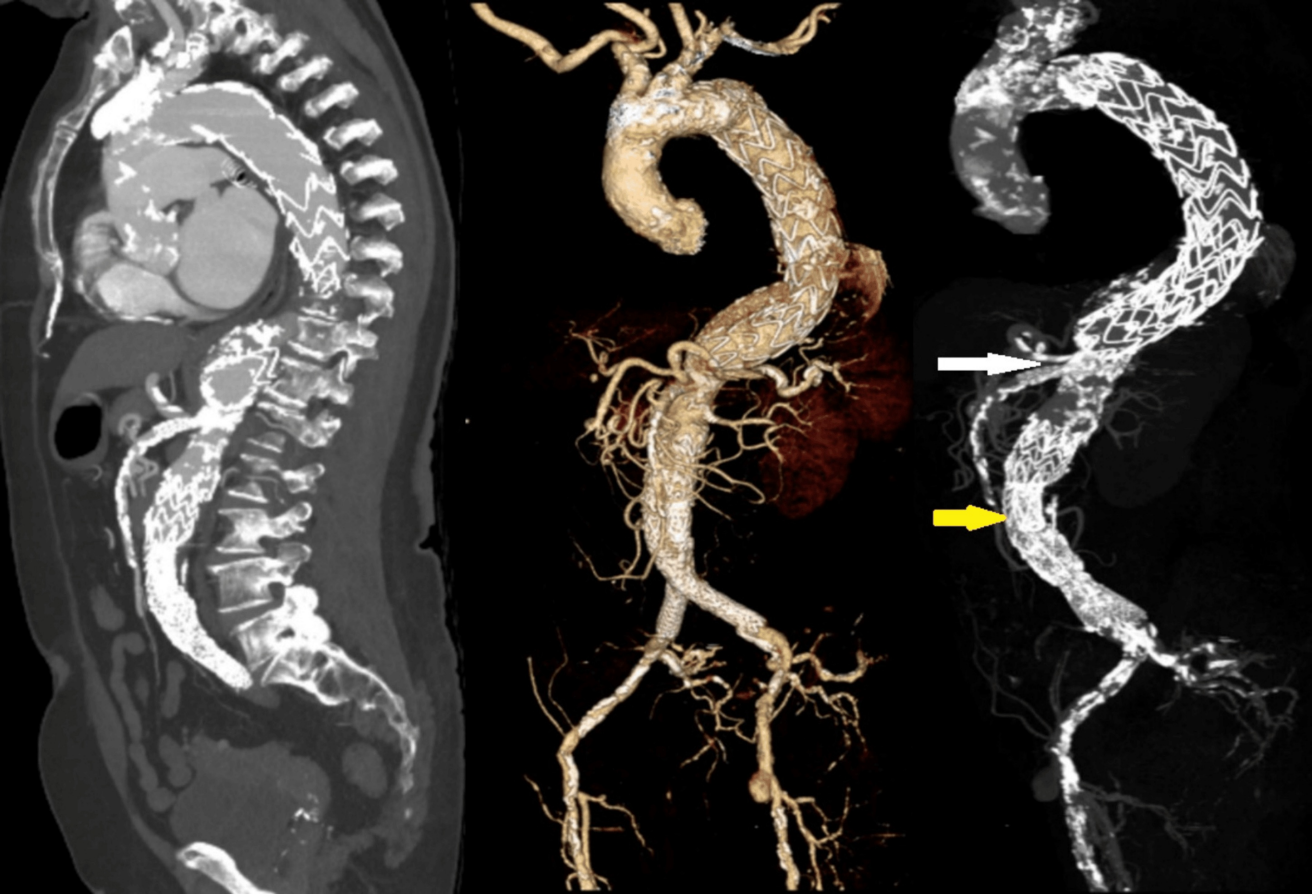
Pre-procedure Computed Tomography Angiography (CTA) of the Chest, Abdomen, and Pelvis The left panel shows a sagittal view of the thoracic and abdominal vasculature. The middle and right panels show the thoracic and abdominal vasculature isolated from surrounding organs and structures. All panels demonstrate a patent descending thoracic aorta, superior mesenteric artery (SMA), and infra-renal aortic stent graft (yellow arrow). Celiac ostium stenosis is re-demonstrated (white arrow), along with extensive abdominal aortic and mesenteric vascular calcification.
